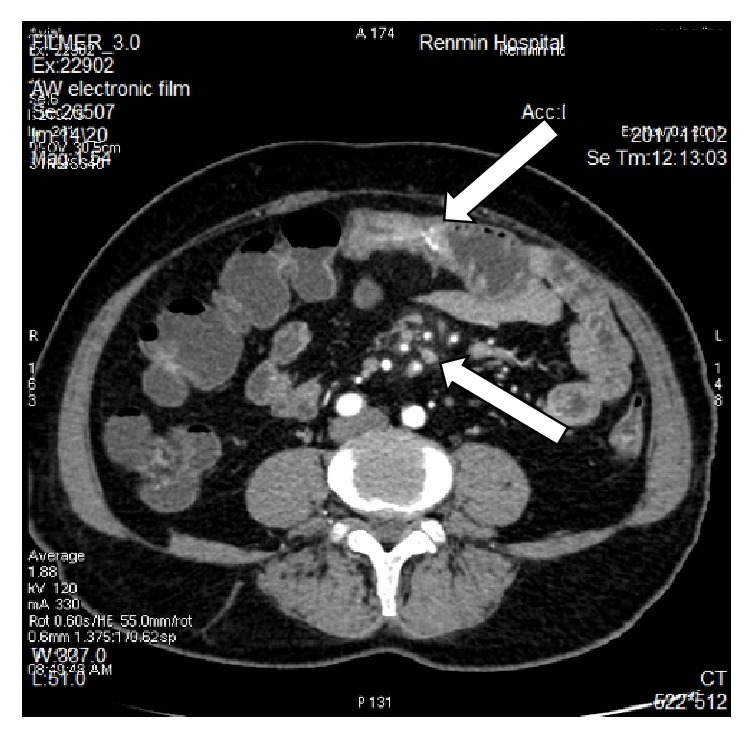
CT scan showing thickened intestinal wall, enlarged lymph nodes.
